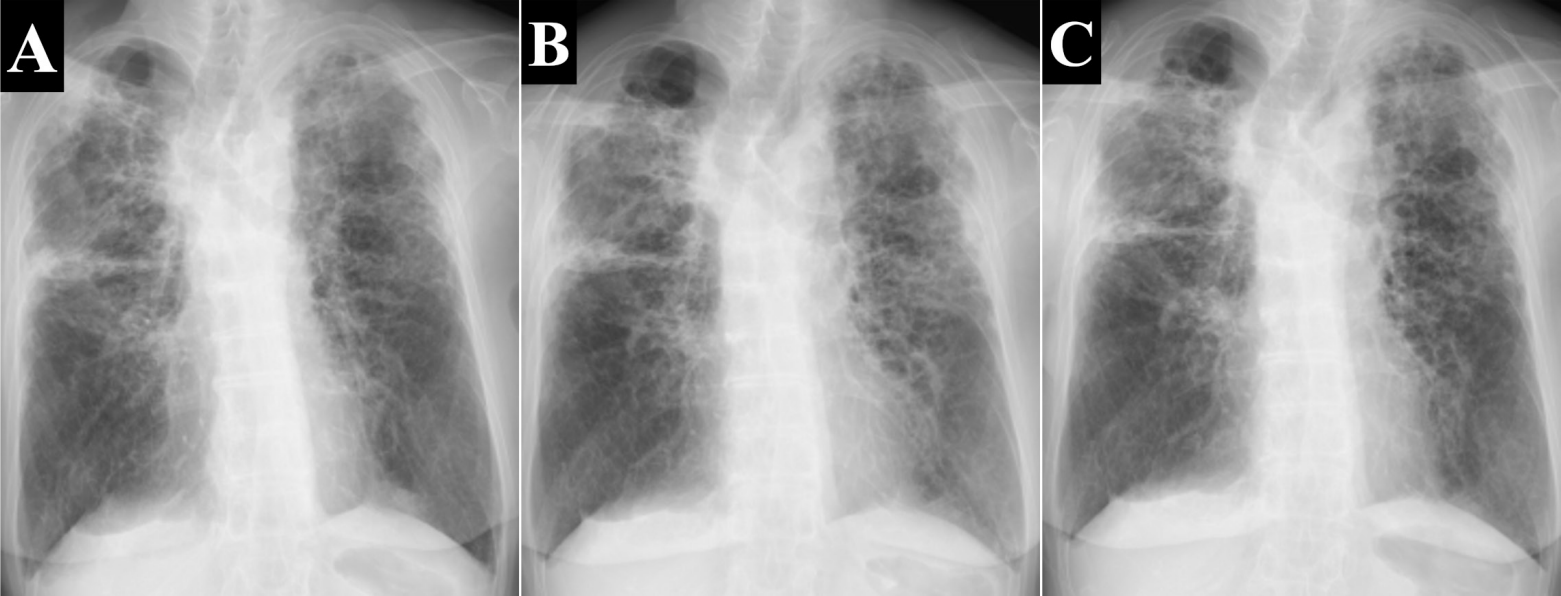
Changes in chest radiographic findings. (A) One year after starting inhaled tobramycin treatment. (B) Three months after discontinuation of inhaled tobramycin, consolidation in the left middle lung field has worsened. (C) One month after resuming inhaled tobramycin, the findings observed during the exacerbation have improved.
